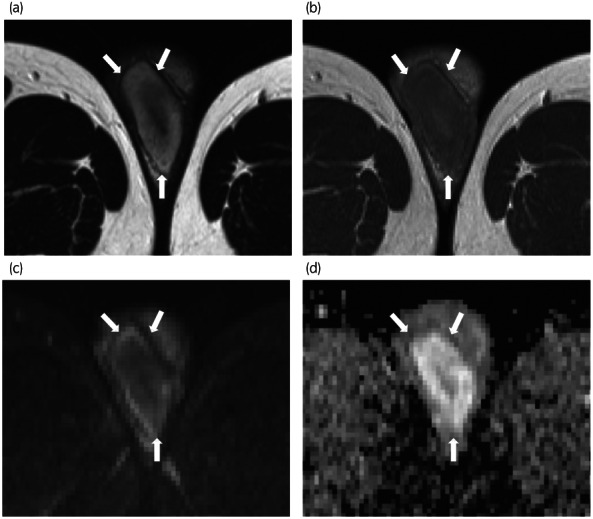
MRI indicating a hypointense lesion at T2 in the center of the right testis (a), and poor contrast effects compared to the contralateral side (b). Diffusion‐weighted imaging confirming hypointensity at the same site (c), and an apparent diffusion coefficient indicating hyperintensity (d).
